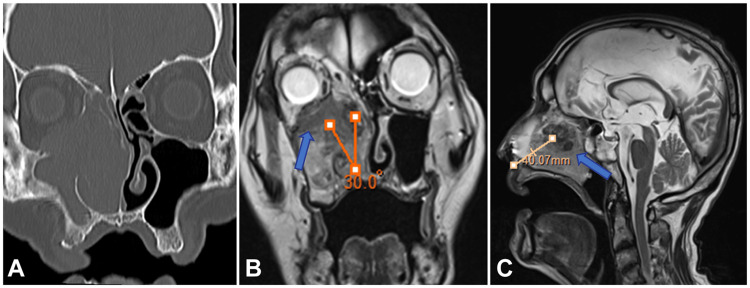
Imaging findings of the paranasal sinuses A) Coronal section sinus CT. B) Coronal T2-weighted MRI image. Low-signal areas indicate areas with suspected malignancy (arrows). The triangular red line indicates the angle from the nasal cavity entrance to the tumor in the medial direction of the orbit. C) Sagittal T2-weighted MRI image. The low-signal area is the site of the suspected malignancy (arrow). The pink line represents the distance from the nasal cavity to the tumor. CT, computed tomography; MRI, magnetic resonance imaging.
